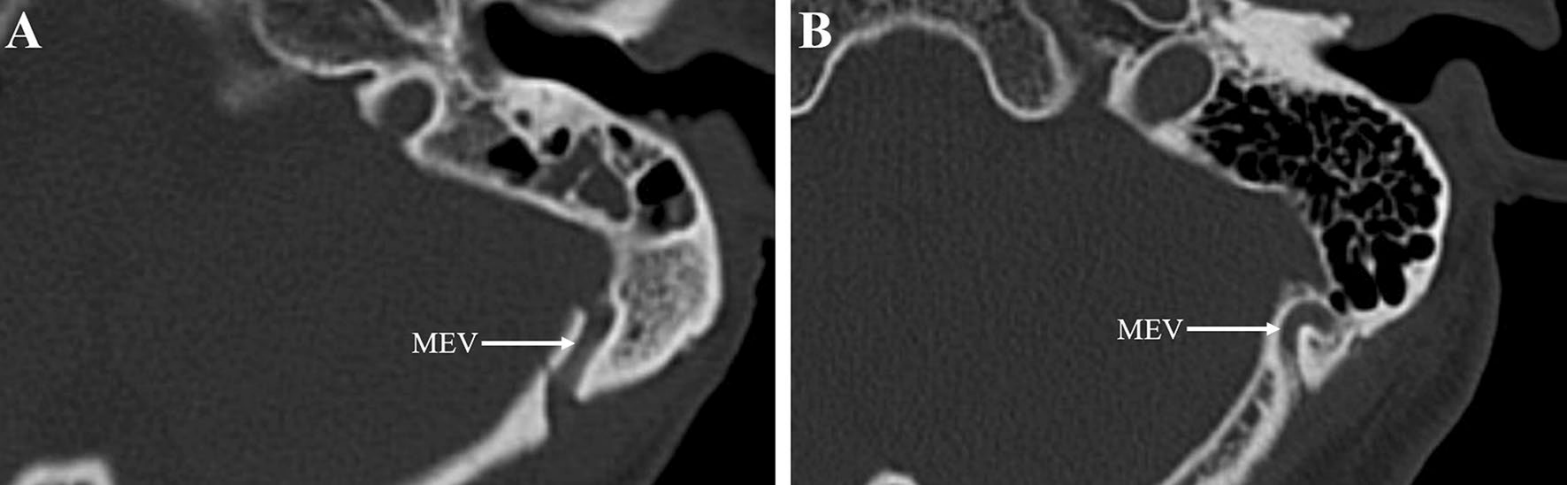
The MEV on CT bone window mode. A Straight type. B Curved type
